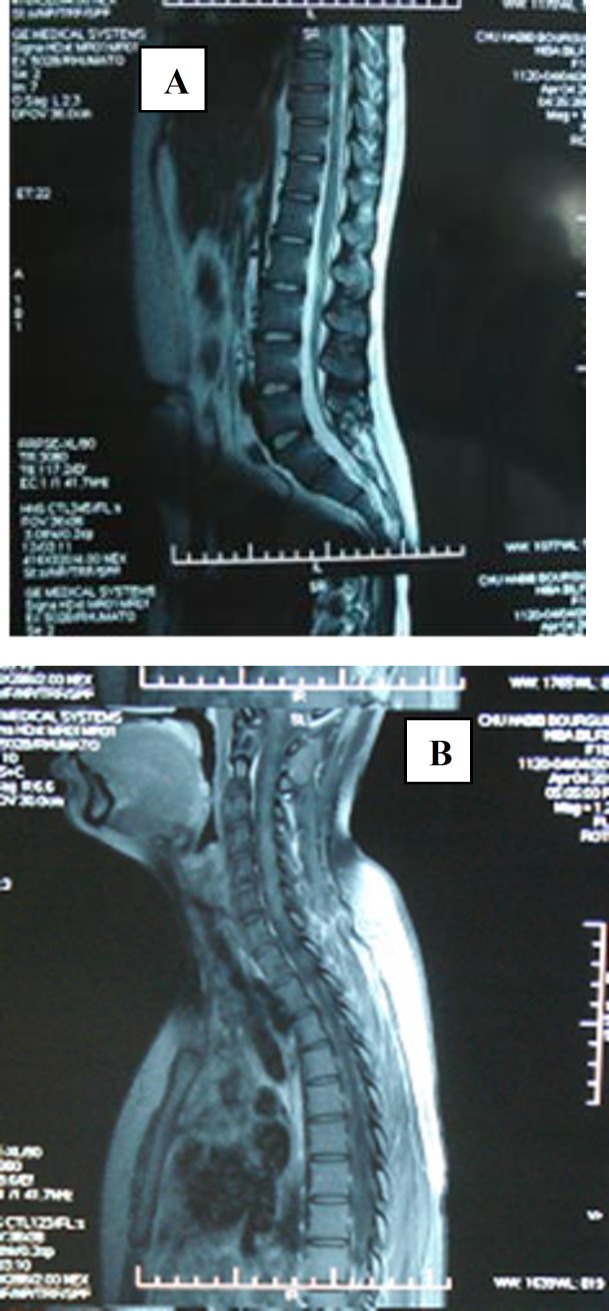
The spinal cord MRI revealed else increased T2 signal extended from the 3^rd^ cervical vertebrae to the 10^th^ thoracic vertebra and from the 1^st^ lumbar to the 2^d^ lumbar vertebrae
